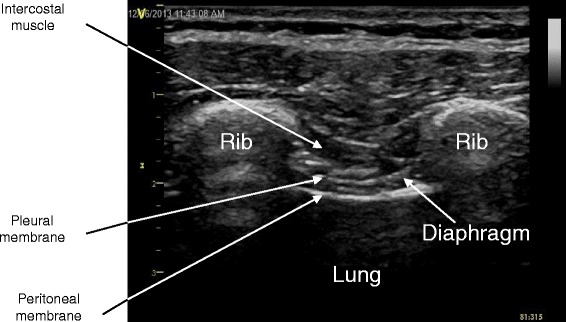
Ultrasound image of normal diaphragm in zone of apposition. A linear array transducer was applied with the use of M-mode ultrasound at the right anterior axillary line at approximately the ninth intercostal space
